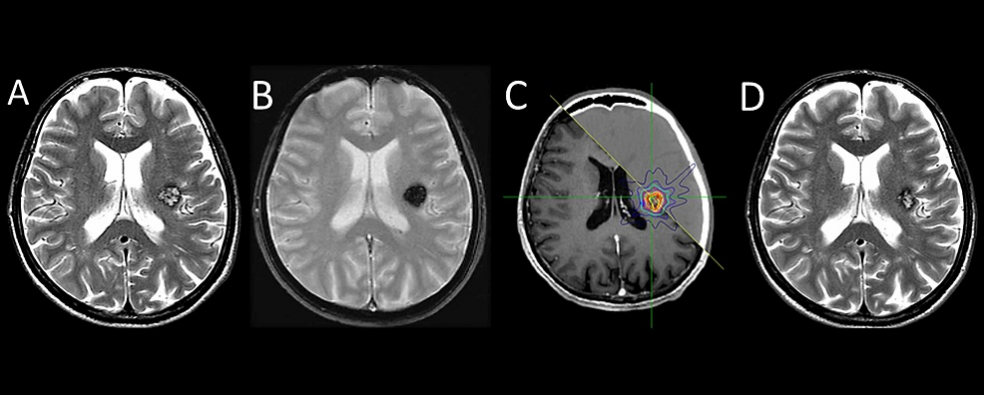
Radiological imaging MRI axial plane, T2-weighted image shows a cavernous hemangioma with peripheral hemosiderin rim at the left posterior insular region (A), MRI axial plane, gradient echo image shows hemorrhagic lesion (B), a fusion of axial planes of MRI and computed tomography shows the target delineation for cavernous hemangioma with isodose distribution (C), MRI axial plane, T2-weighted image shows a considerable decrease in the size of cavernous hemangioma in 30 months follow-up after CyberKnife (D).
